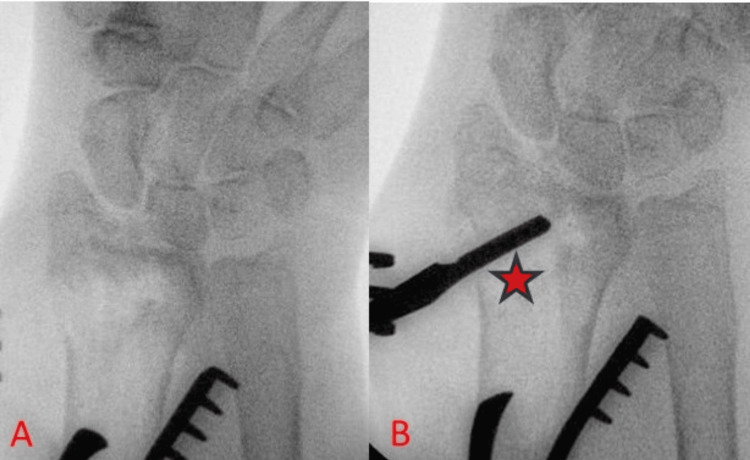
Reduction technique for die-punch fragments (A) Intraoperative anteroposterior radiograph showing a displaced die-punch fragment of the distal radius. (B) Intraoperative radiograph showing the introduction of a dissector (noted with red asterisk) through the fracture window, elevating the die-punch fragment in line with the rest of the articular surface.
